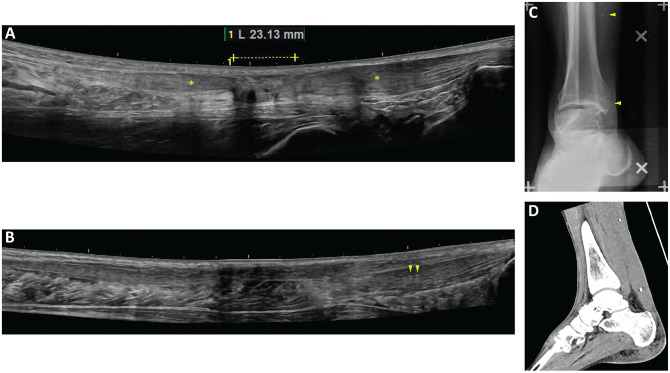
(A) Ultrasound image from an injured Achilles tendon indicating the injured hypoechogenic area (dotted line) and areas with a well-organized linear collagen structure where the proximal and distal beads are injected (asterisks). (B) Ultrasound image from a healed tendon injury (4 years after the injury). The distal beads are visible and indicated by arrowheads. Images from (C) roentgen stereophotogrammetric analysis (beads indicated by arrowheads) and (D) computed tomography (1 year after the injury).
